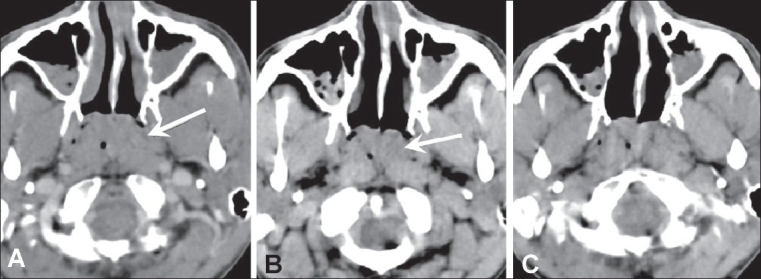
Contrast-enhanced CT scan images shows a moderately enhancing mass (arrow) extending up to the posterior choanae
